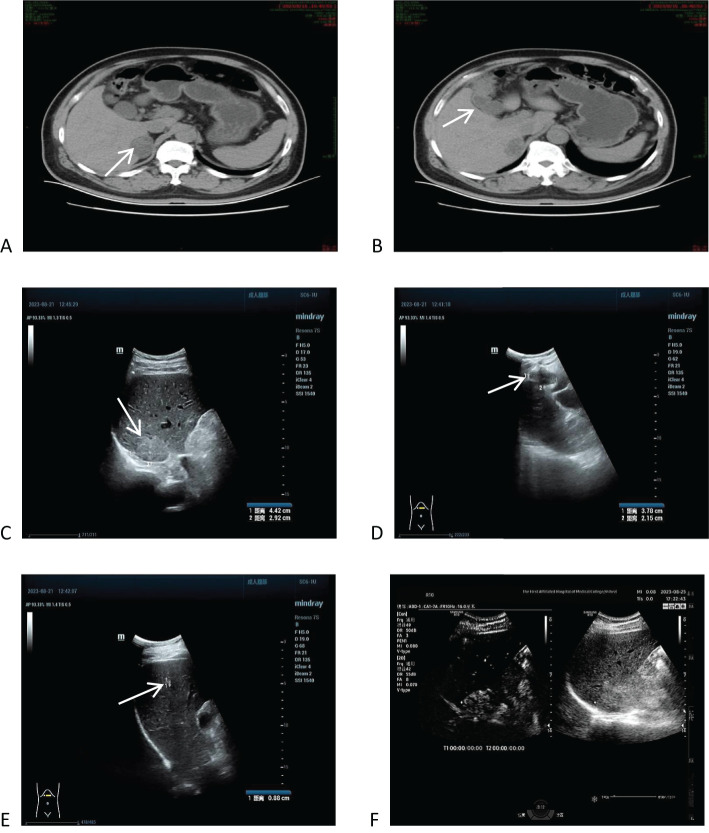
Computed tomography (CT) and ultrasound imaging of the upper abdomen, and ultrasonographic findings. (A) CT images showed a 3.8cm × 4.1cm × 2.9cm mass of hypodense opacity in the right adrenal region. (B) The bottom and wall of the gallbladder were obviously thickened and rough, with significant enhancement on the enhancement scan. (C) Ultrasound showed that there was a hypoechoic area with a size of about 4.4cm × 2.9 cm between the liver and the kidney. (D) One of the slightly elevated echoes was seen in the liver, which was 0.8cm × 0.7cm. (E) Ultrasonography shows a “fast in, fast out” pattern in the right lobe of the liver and a hypoechoic area at the gallbladder floor. (F) Further contrast-enhanced ultrasound showed high suspicion for hepatocellular carcinoma (HCC) and gallbladder carcinoma based on contrast agent dynamic changes.
